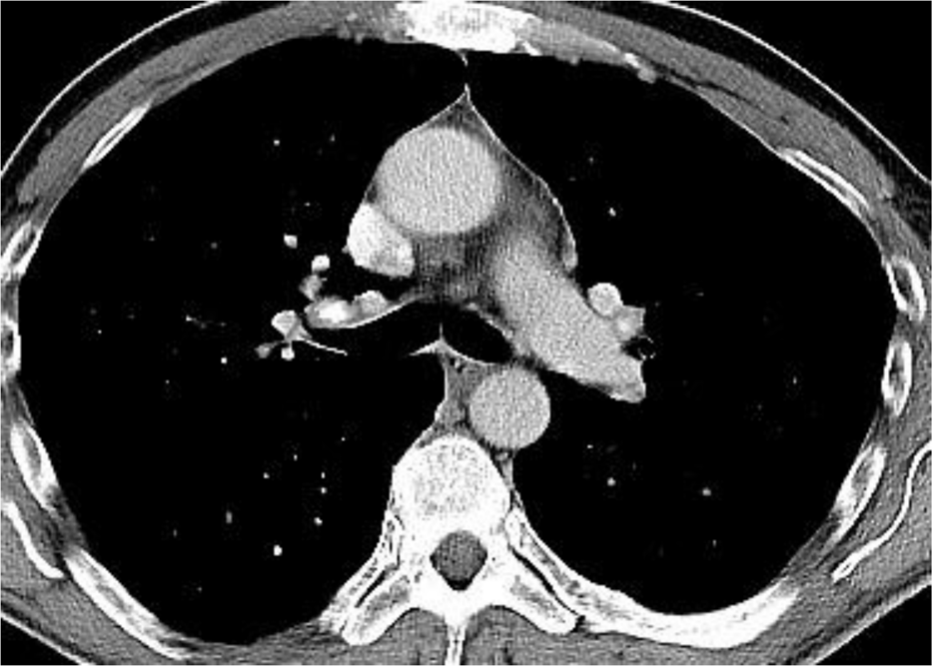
CT Thorax showing mediastinal lymphadenopathy.
